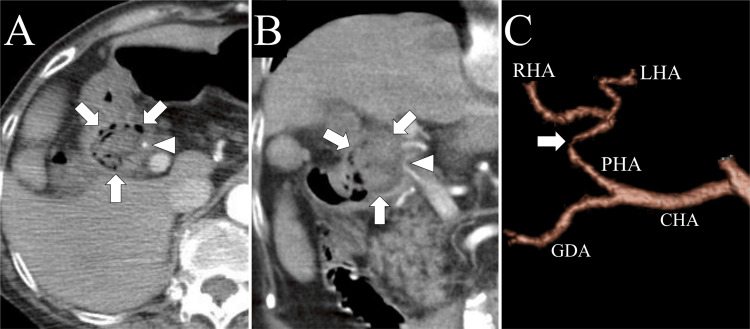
Contrast-enhanced computed tomography (CT) at presentation. Axial (A) and coronal (B) arterial-phase contrast-enhanced CT images show a duodenal ulcer (arrows) at the duodenal bulb. The proper hepatic artery (arrowheads) is observed at the base of the ulcer.
